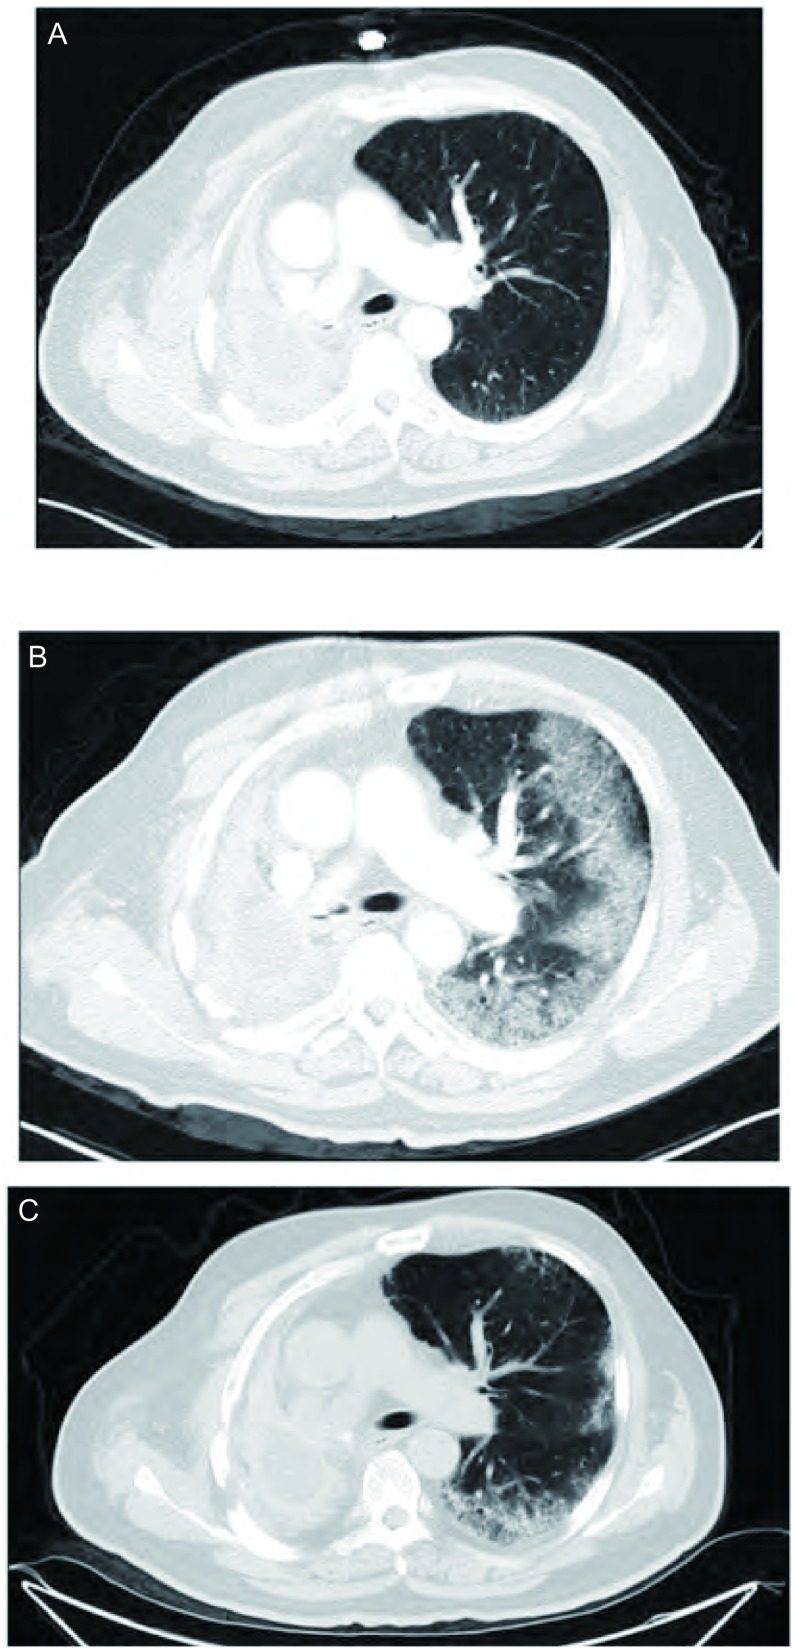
病例4厄洛替尼治疗前后及糖皮质激素治疗后胸部CT变化 Chest CT scans before and after erlotinib treatment and after corticosteroid treatment. A: Chest CT scan before erlotinib treatment; B: Chest CT scan after 22 days of erlotinib treatment; C: Chest CT scan after 10 days of corticosteroid treatment.
